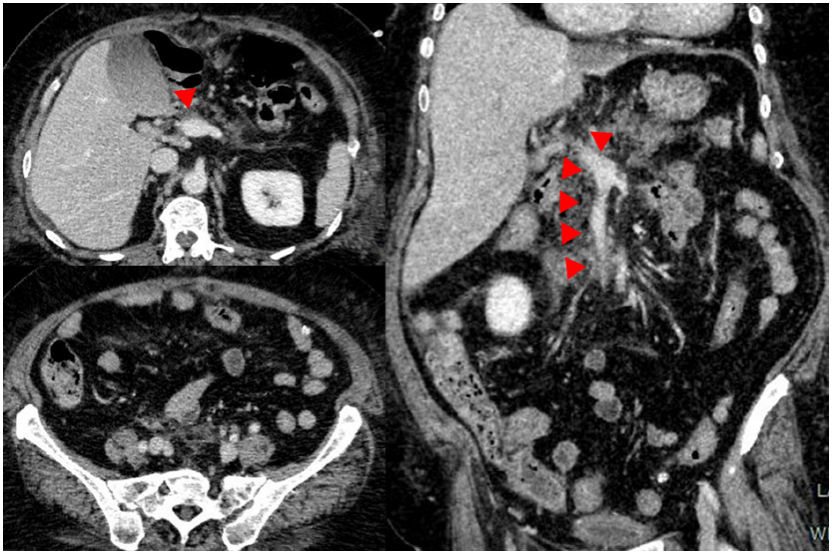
Fig. 3 Postoperative computed tomography of the abdomen and pelvis. The superior mesenteric venous thrombi is resolved (arrowheads), and the edematous bowels have improved.
